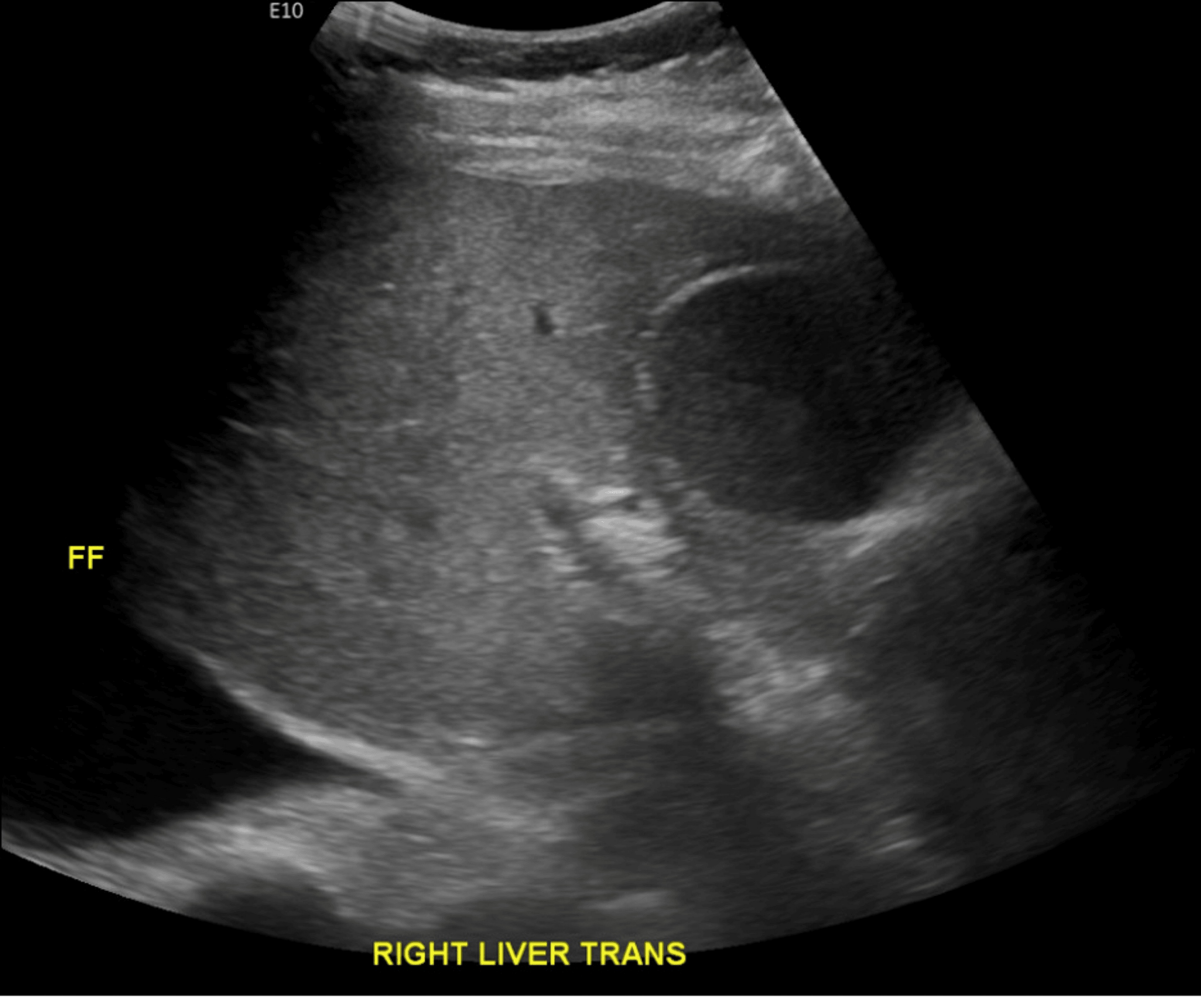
US abdomen US: ultrasound; FF: Free fluid noted Sludge is seen within the gallbladder. No discrete shadowing stones are seen. Thickened gallbladder wall measuring 4 mm. No pericholecystic fluid. Positive Murphy sign
